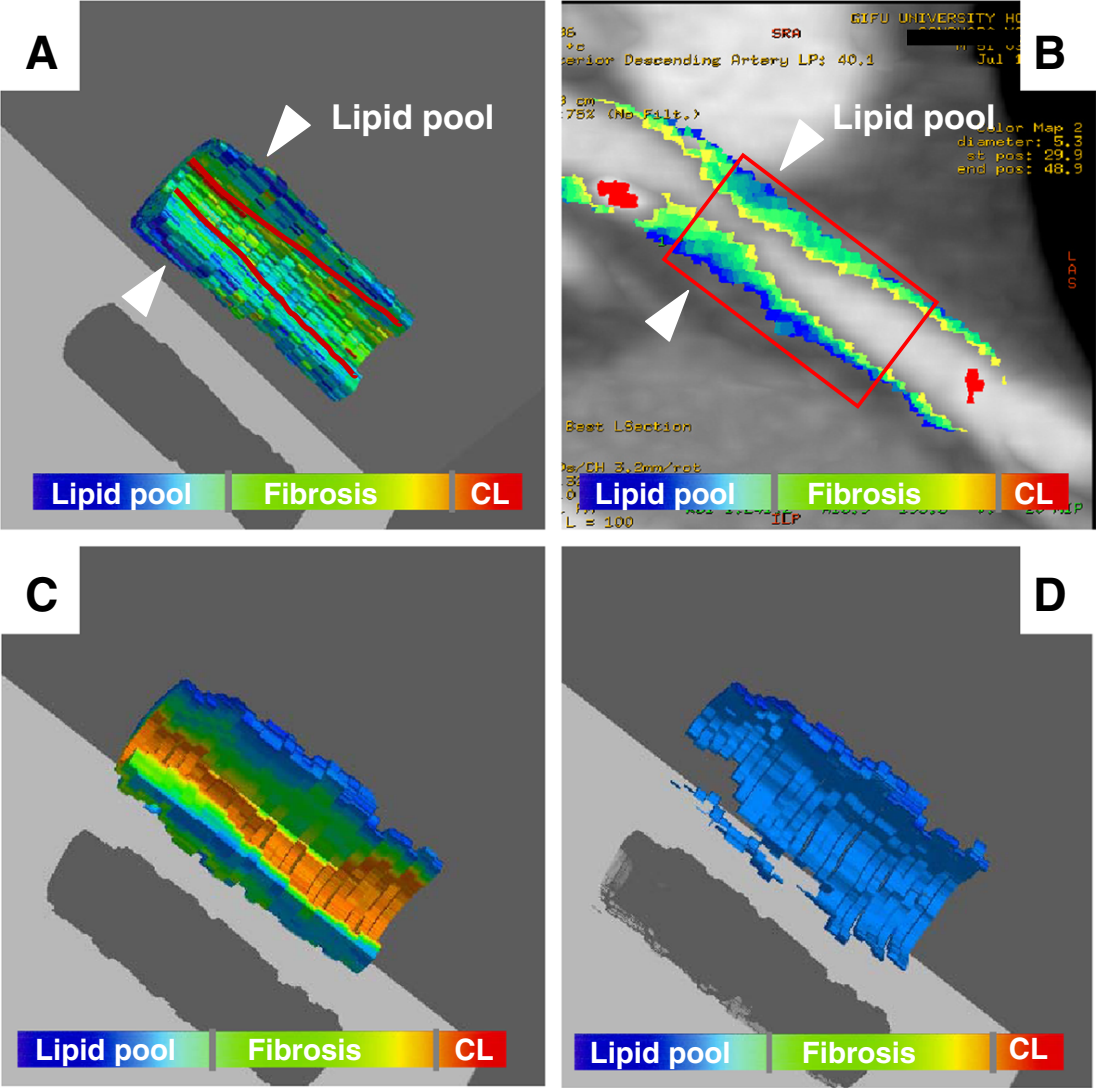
Representative case of three-dimensional (3D) color-coded maps (68 years old, male). To reconstruct 3D IB-IVUS color-coded images, the IB-IVUS images were transformed into Cartesian coordinates (64 x 64 pixels) using customized computer software and compared with MDCT images in the Cartesian coordinates (256 x 256 pixels). We then manually excluded the vessel lumen and area outside of the intima in both the 2D IB-IVUS and MDCT images. Three dimensional constructions were automatically performed by computer software (T3D, Fortner Research LLC, Sterling, Virginia). (A) 3D color-coded map constructed by integrated backscatter intravascular ultrasound of coronary plaque with intermediate stenosis. (B) Color-coded curved multiplaner reconstruction image of coronary plaque. (C) 3D color-coded map constructed by multidetector computed tomography for the same lesion as in (A). (D) 3D color-coded map constructed by multidetector computed tomography that showed only lipid pool in the coronary plaque that was same lesion as (C).
